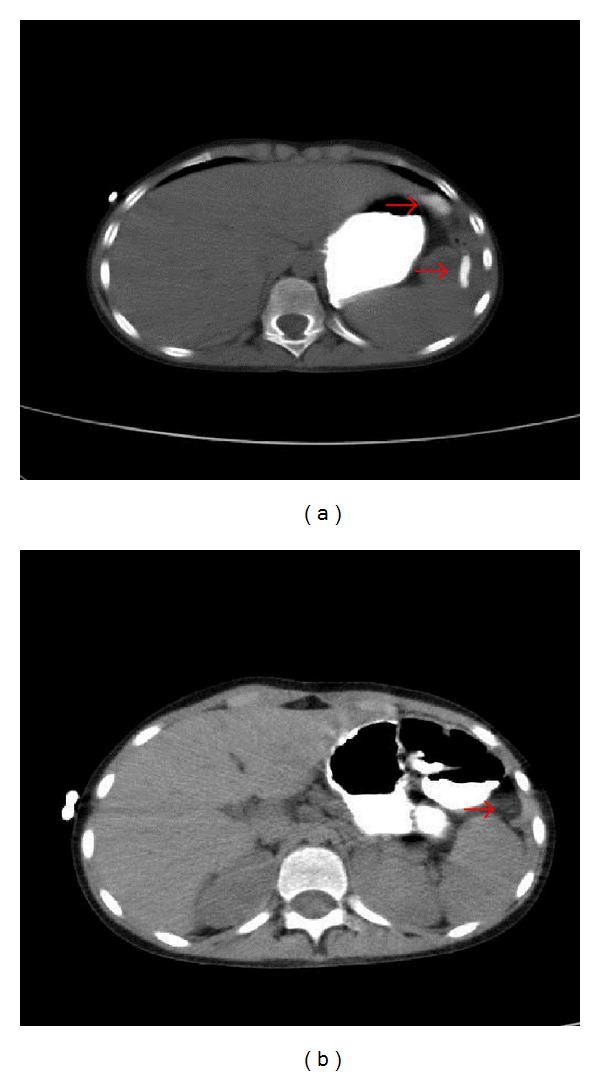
Axial abdominal CT projections (with enteric contrast enhancement) showing a progressive healing of the fistula connecting the colonic lumen to the collection before (a) and after (b) the second administration of anti-TNF-*α*.  Figure 2(a). detects the passage of enteric contrast from the colon into the parietal-colonic collection, which is no more visible in Figure 2(b).
